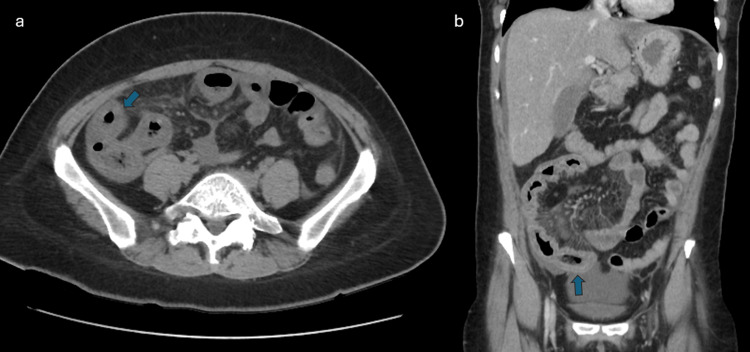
First contrast-enhanced computed tomography (CT) scan of the abdomen and pelvis In the axial (a) and coronal sections (b), we can see significant diffuse thickening of the distal ileum (arrows).
